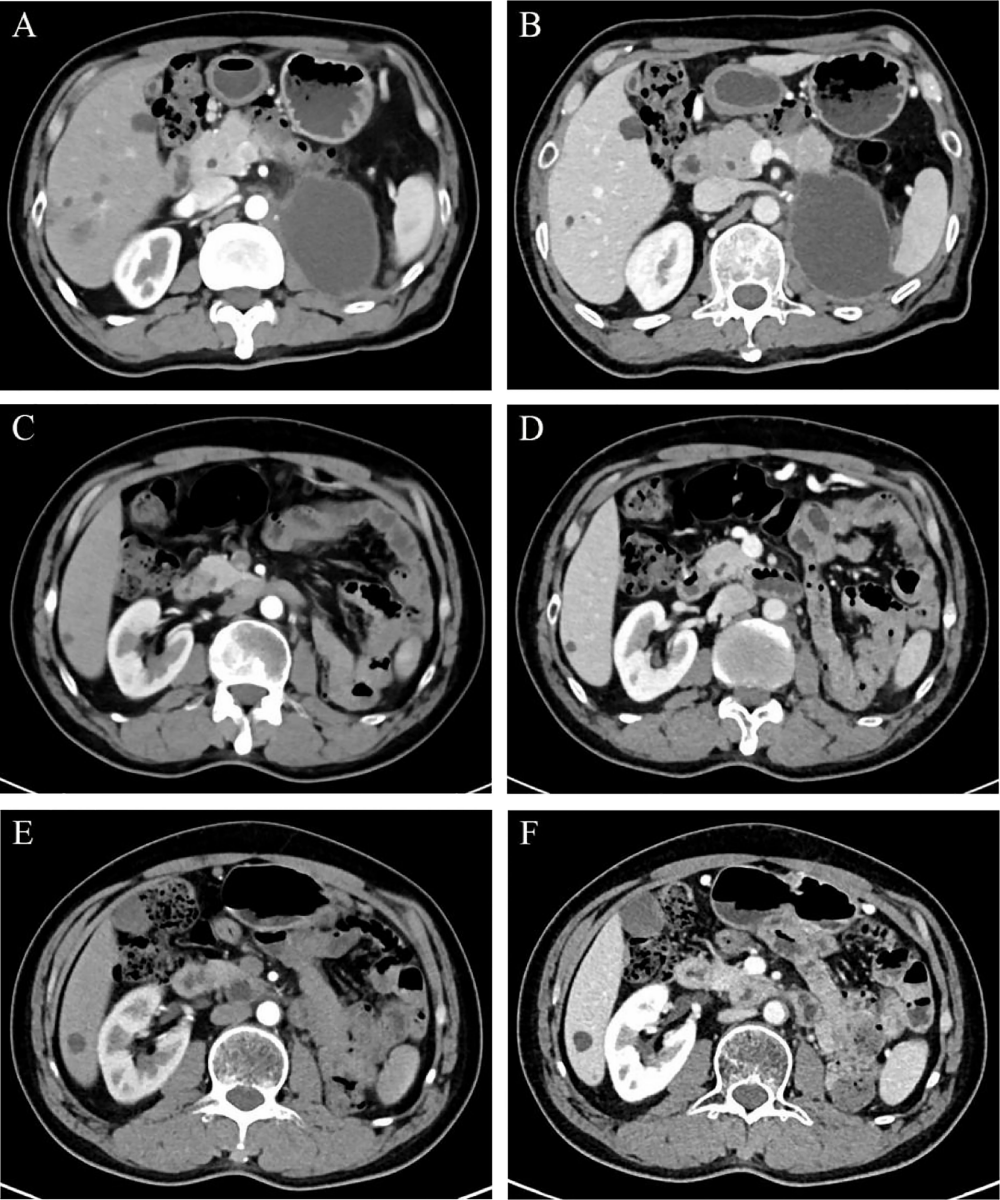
Regular dynamic contrast-enhanced CT scan manifestations in CMP (A, C, E) and NP (B, D, F). (A, B on July 24, 2020) An 8.0 × 5.7 cm cystic low-density lesion was found in the original left renal area. (C, D on August 2, 2021) The cystic effusion was absorbed completely. (E, F on August 17, 2022) The original left kidney area was filled with intestines. Abbreviations: CMP, corticomedullary phase; NP, nephrographic phase.
